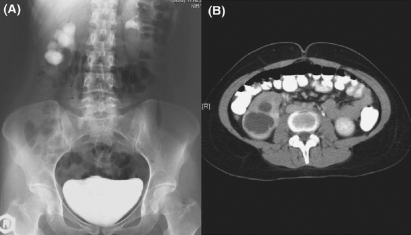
(A) IVU showing a dilated lower calyx. (B) CT scan showing a cavity in the lower pole, non-communicating with the rest of the collecting system
